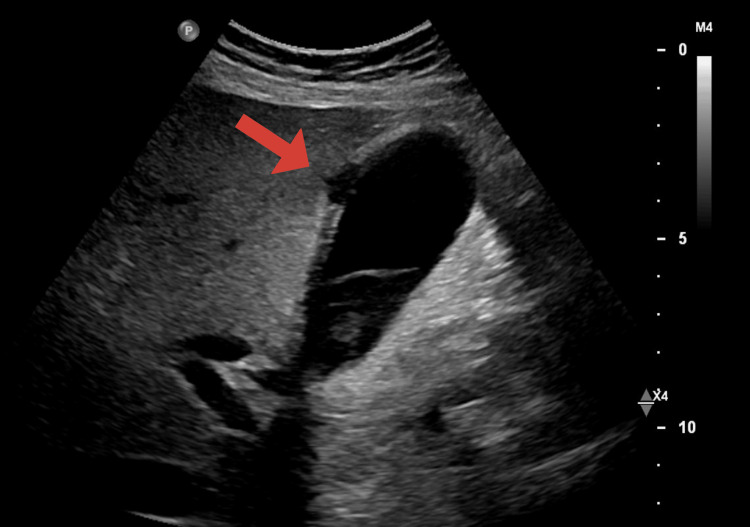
Gallbladder ultrasound Findings were concerning for acute gangrenous cholecystitis with focal intrahepatic perforation along the gallbladder fossa of the liver. There was increased conspicuity of the pericholecystic fat.
